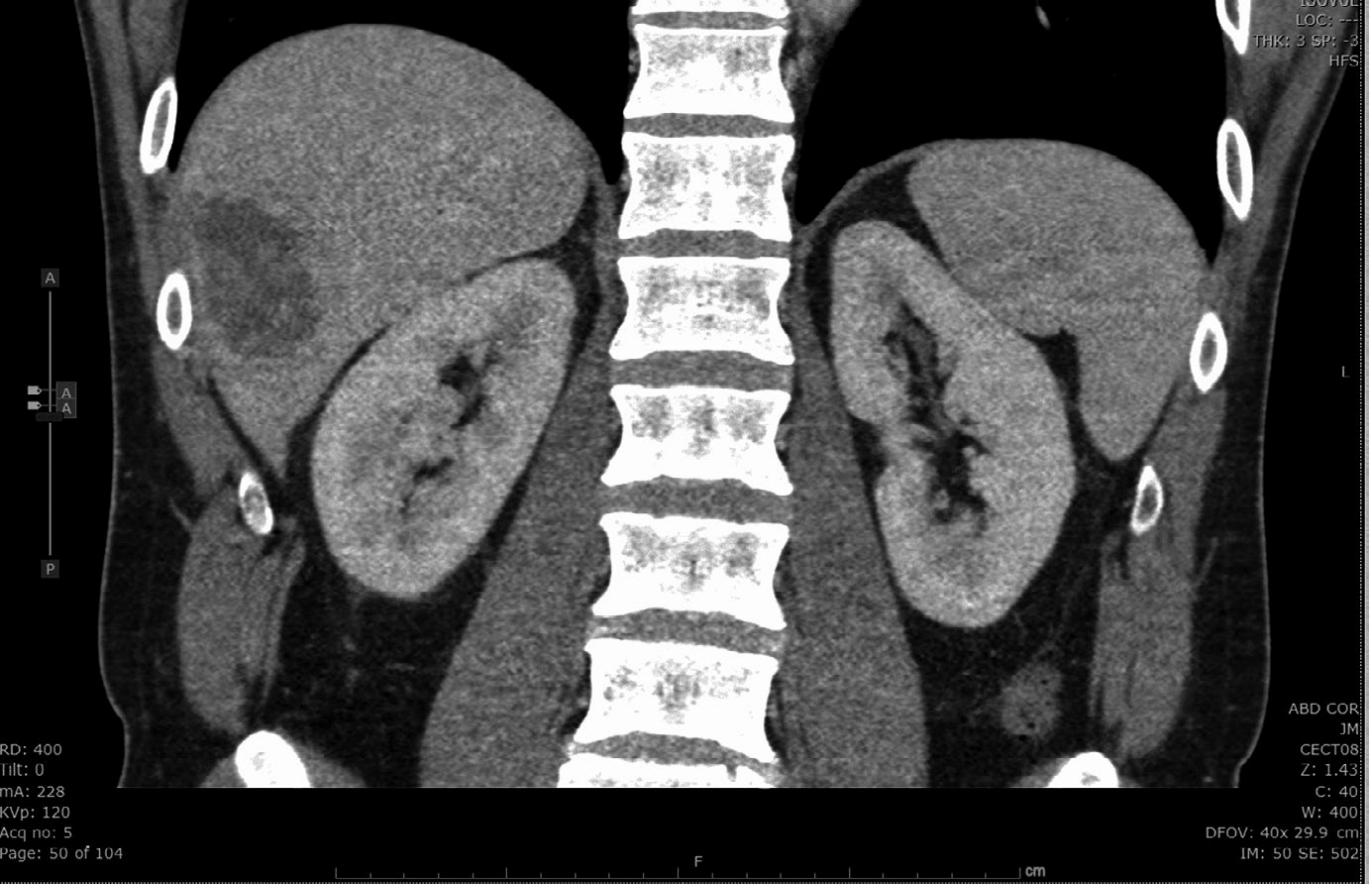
Computed tomography (CT) scan, during hospitalization, showing multiseptated complex larger mass with thick wall and internal fluid attenuation, measuring 5.5 × 3.8 × 5.2 cm in size
